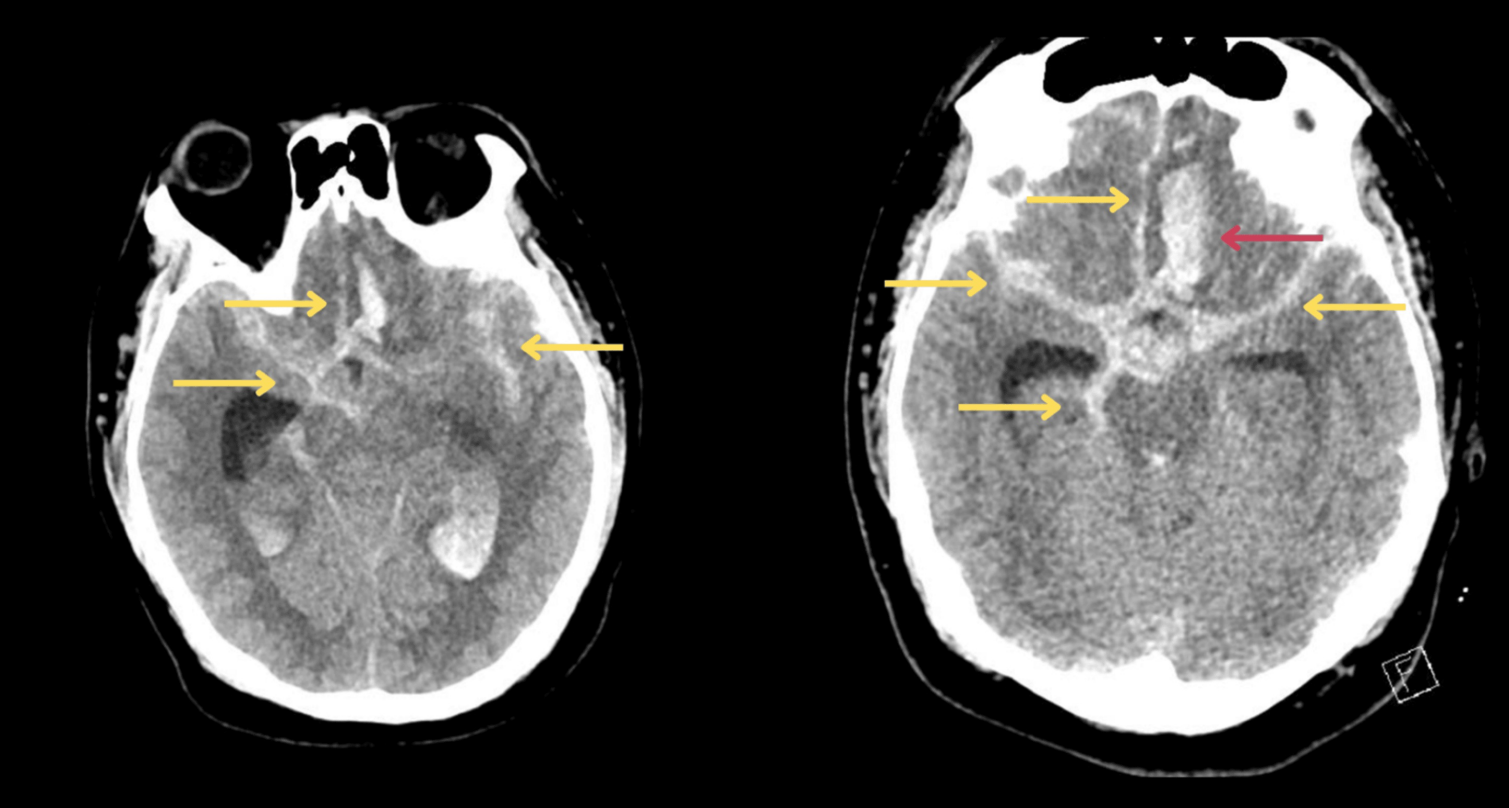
Plain cranial CT scan done one hour post-ictus showing diffuse subarachnoid hemorrhage (yellow arrows) with intracerebral hemorrhage (red arrow) CT: computed tomography
